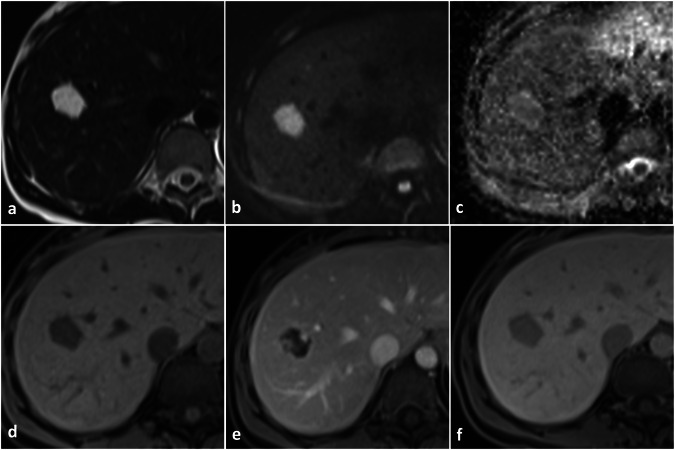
Typical MRI findings in cavernous hemangioma. Liver hemangioma appears markedly hyperintense on T2-weighted images (a) determining shine through artifact on high b value DWI (b) without true diffusion restriction on ADC map (c). The lesion appears hypointense on T1-weighted images (d), shows globular peripheral enhancement in the arterial phase (e) and appears hypointense in the hepatobiliary phase (f)
